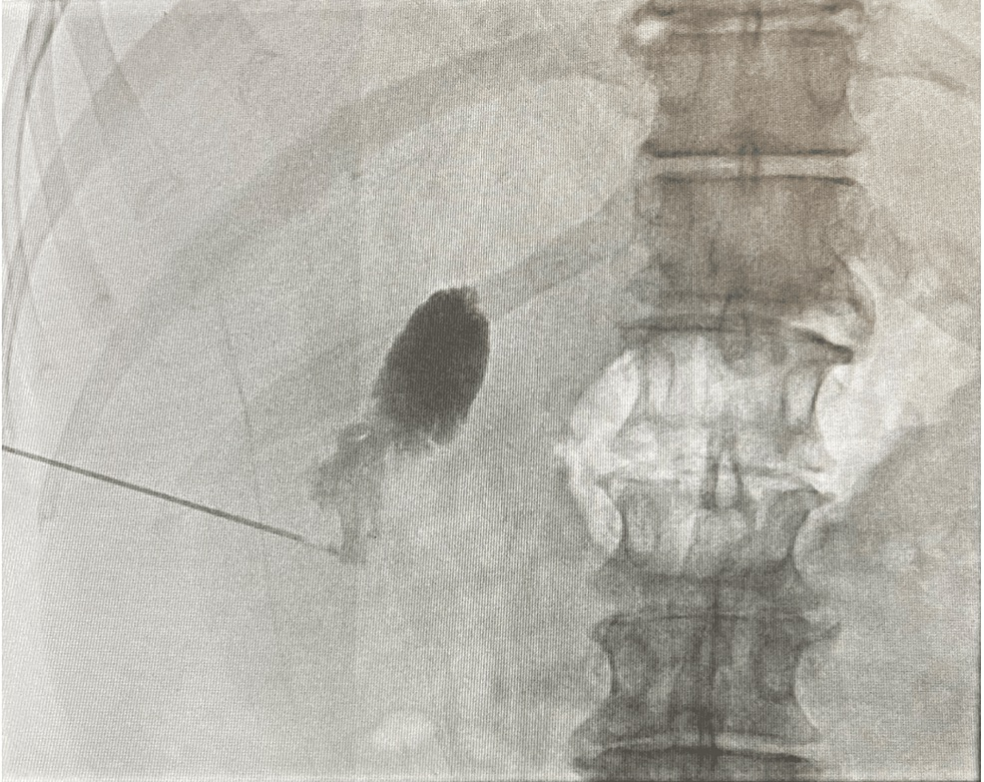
CT-guided cholecystostomy tube (IR)
